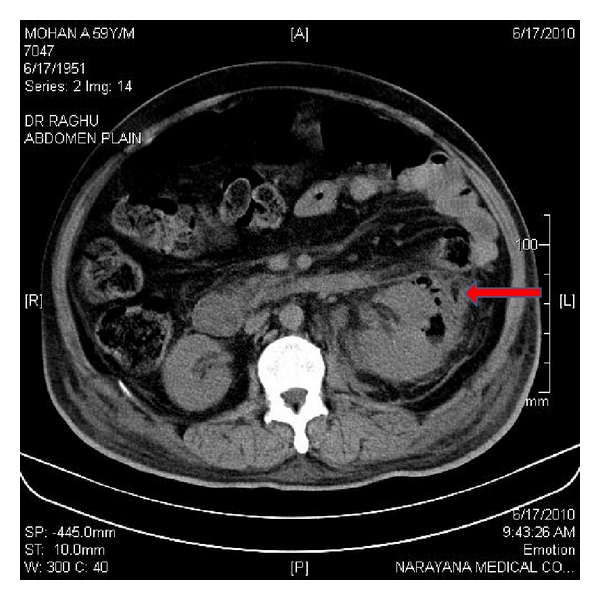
CT KUB showing air pocket in left renal parenchyma and perinephric collection.
